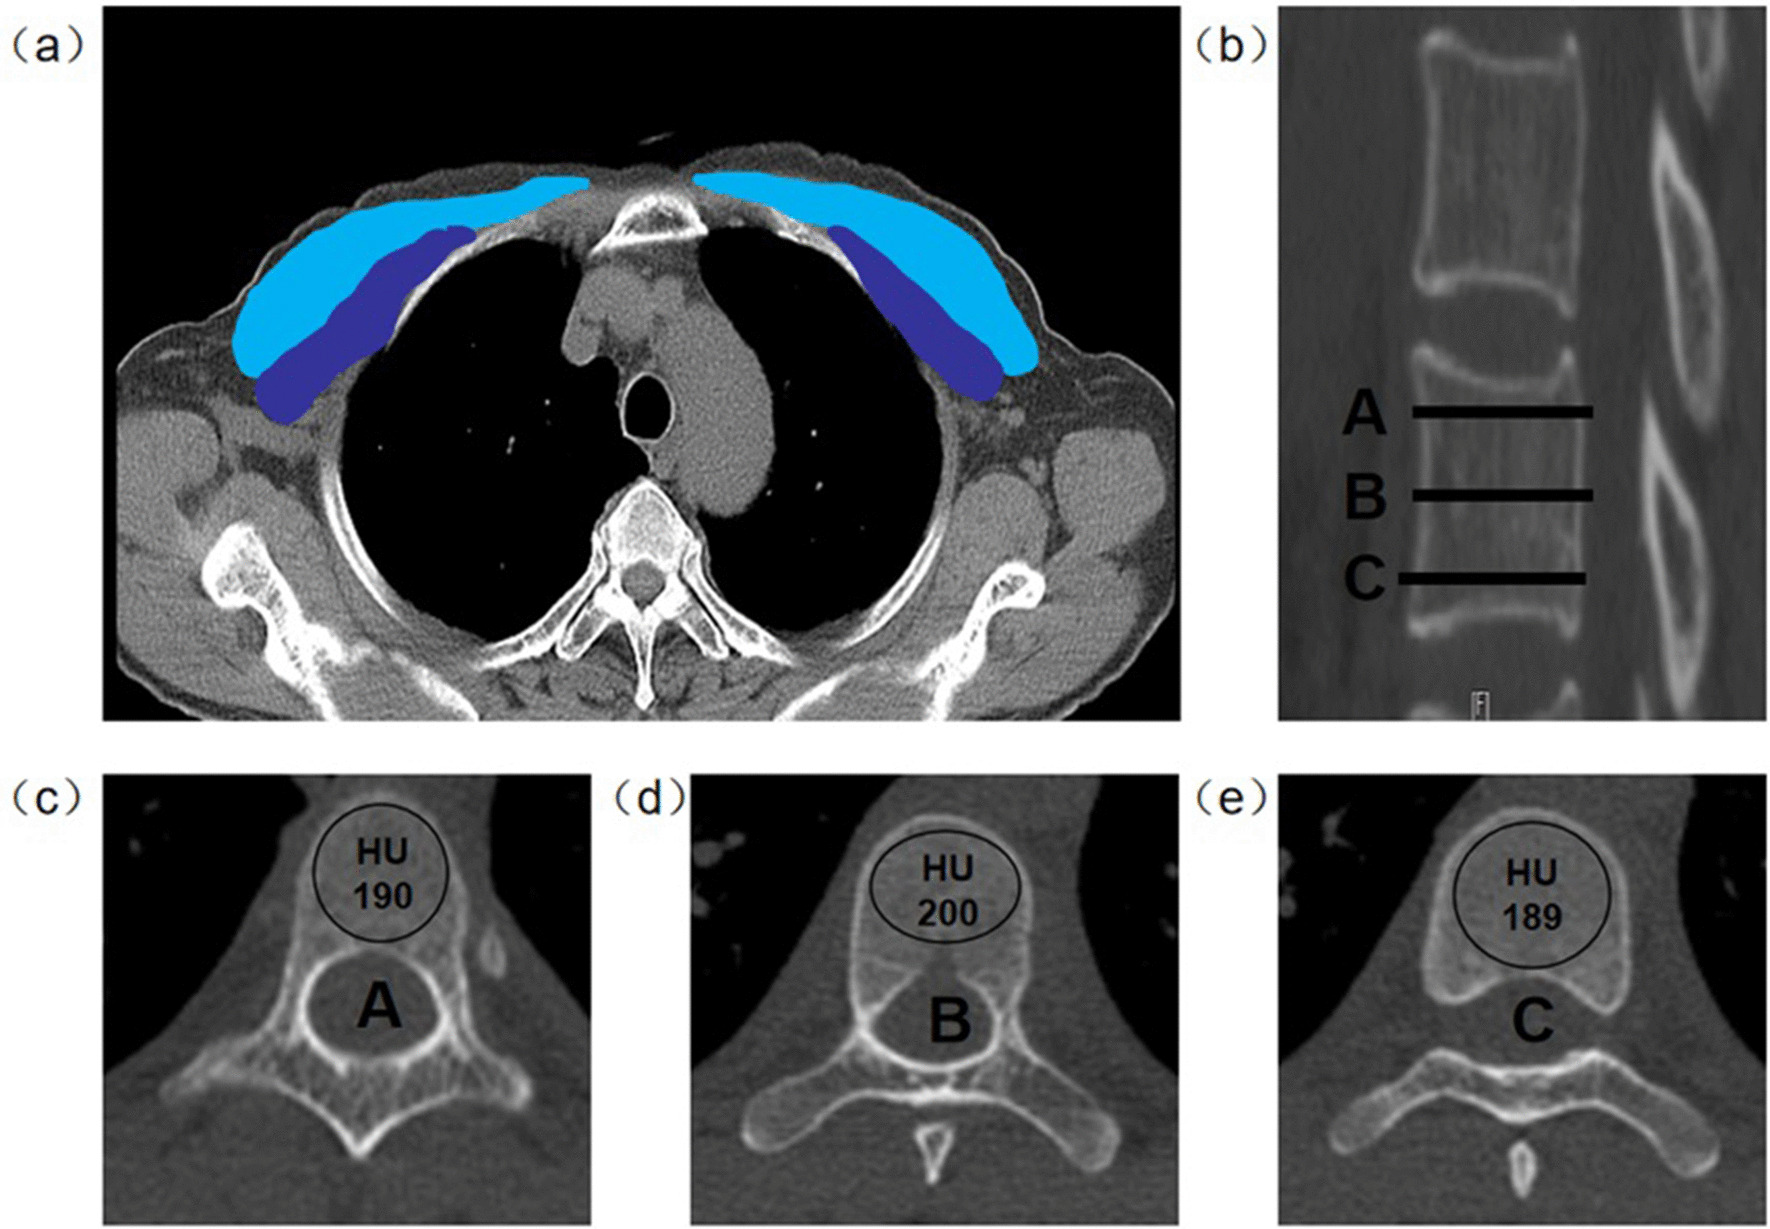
Method for determining the PMA and average vertebral HU values using CT. a Horizontal plane of the sternal angle; the pectoralis major is blue, and the pectoralis minor is purple. b Vertebral body CT sagittal plane. c–e CT scan of three levels of a single vertebra in the sagittal plane (A, near the upper end plate; B, middle of the vertebrae; C, near the lower end plate). CT, Computed tomography; PMA, Pectoralis muscle area
